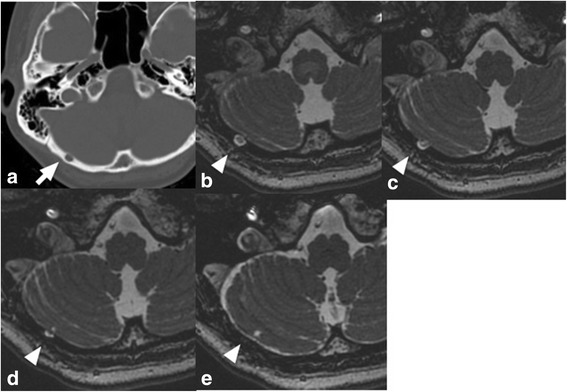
Non-contrast computed tomography via a bone window reveals an oval bone defect in the right occipital bone (a, *arrow*), and features shown by magnetic resonance imaging using constructive interference in steady state axial sequences (b, c, d, e, *arrowheads*)
